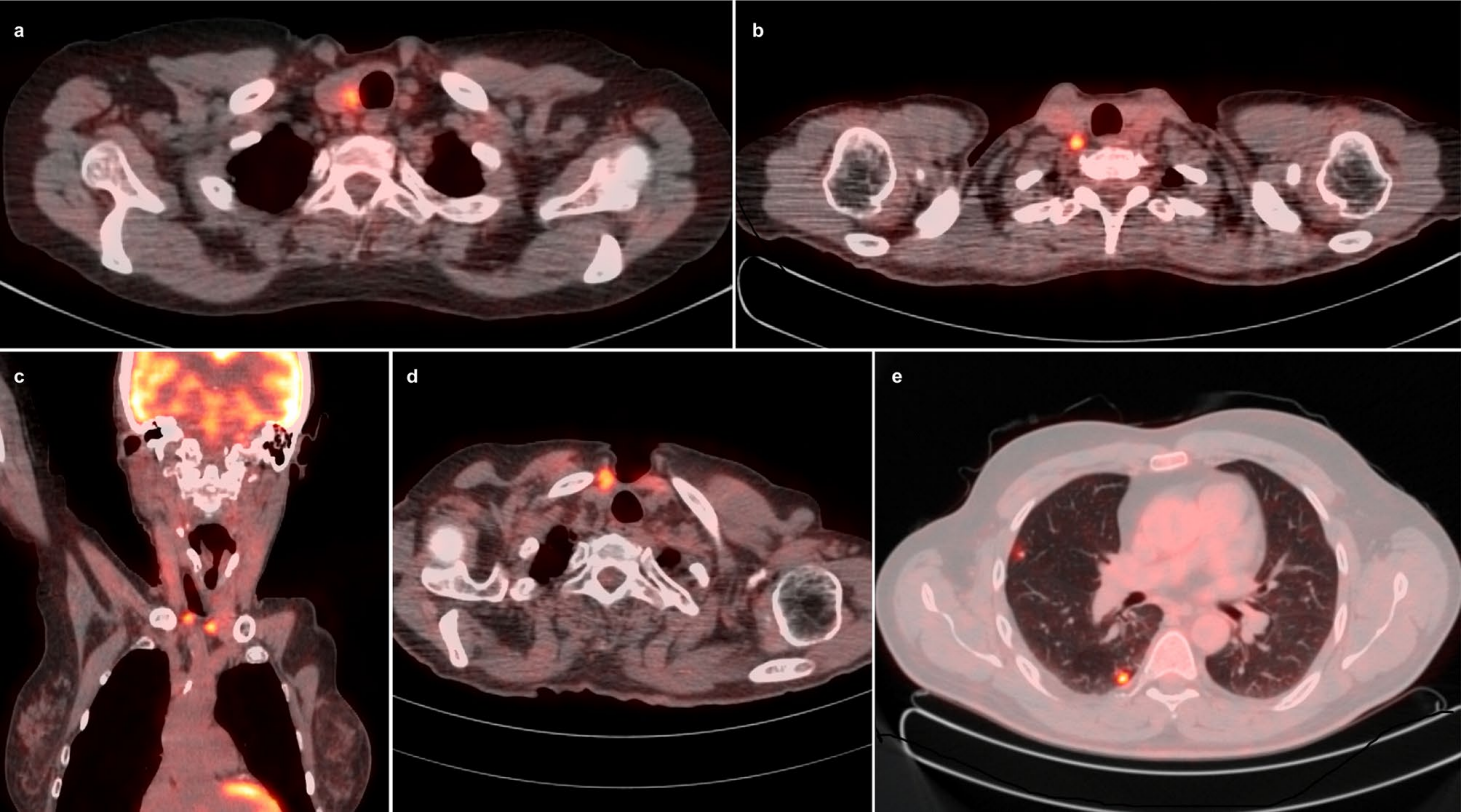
Examples of [^18^F]FDG PET/CT scans performed in patients with TENIS syndrome. (a) 70-year-old woman with papillary thyroid carcinoma with thyroglobulin level 17.28 ng/ml. PET scan detected an [^18^F]FDG-avid metastatic lesion in the thyroidectomy bed. (b) A 61-year-old woman with papillary thyroid carcinoma and thyroglobulin level 4.03 ng/ml. PET scan showed [^18^F]FDG-avid metastasis to the lymph node in the lateral neck compartment. (c, d) A 73-year-old woman with thyroid follicular carcinoma and thyroglobulin level 53.81 ng/ml. PET scan showed [^18^F]FDG-avid recurrent foci in subcutaneous tissue and muscles. (e) A 42-year-old man with thyroid follicular carcinoma and thyroglobulin level 202.60 ng/ml. PET scan detected [^18^F]FDG-avid lung metastases
